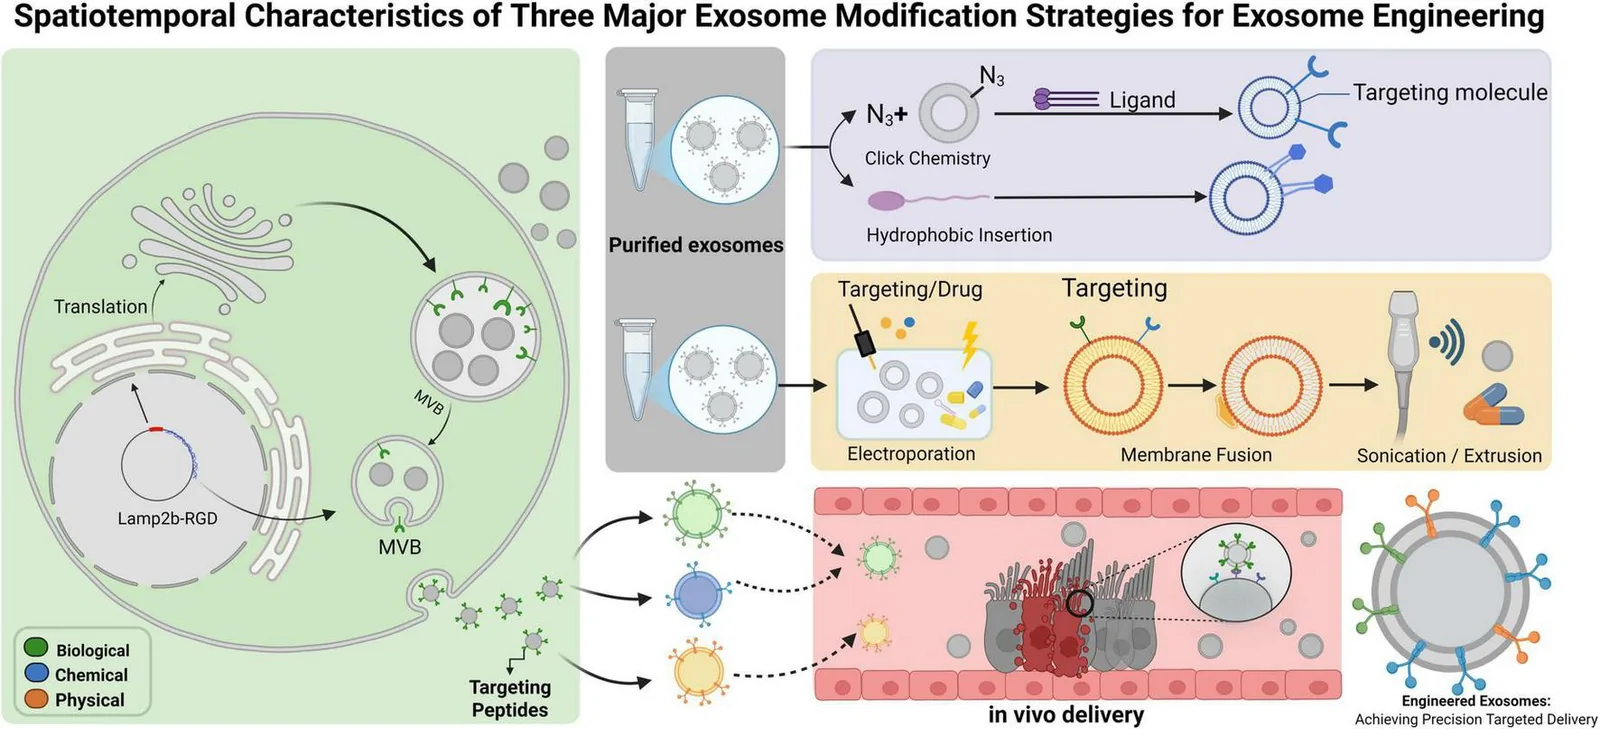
Spatiotemporal characteristics of three major exosome modification strategies for exosome engineering. Three major modification strategies for engineered exosomes are: biological modification, chemical modification, and physical modification. Biological modification is primarily completed within donor cells, allowing the display of targeting peptides or membrane proteins on the exosome surface through genetic engineering; chemical modification is mostly done following exosome isolation and can connect targeting molecules through click chemistry, hydrophobic insertion, or covalent conjugation; physical modification loads drugs or functional molecules via electroporation, membrane fusion, ultrasound, or extrusion. The three strategies differ in operation stages and modification methods; however, they all aim to improve the targeted delivery capacity of exosomes. Green, blue, and orange represent biological, chemical, and physical modifications, respectively. The figure was created with BioRender.com.
